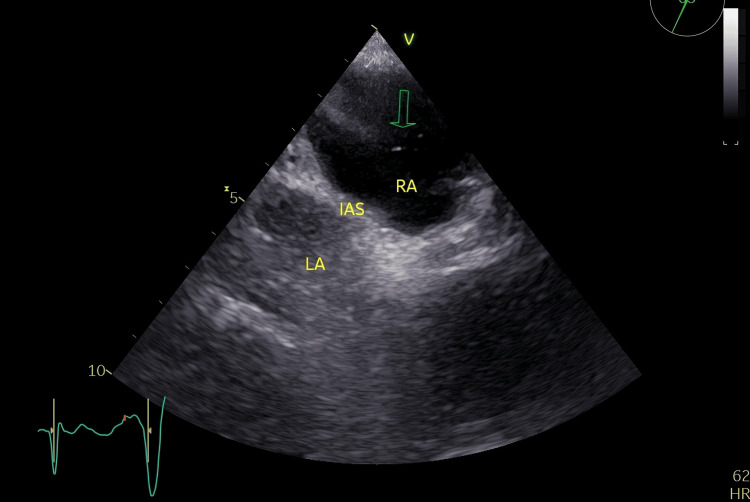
Contrast-enhanced echocardiography Presence of bubbles in the left heart chambers after the fourth cardiac cycle (arrow)
